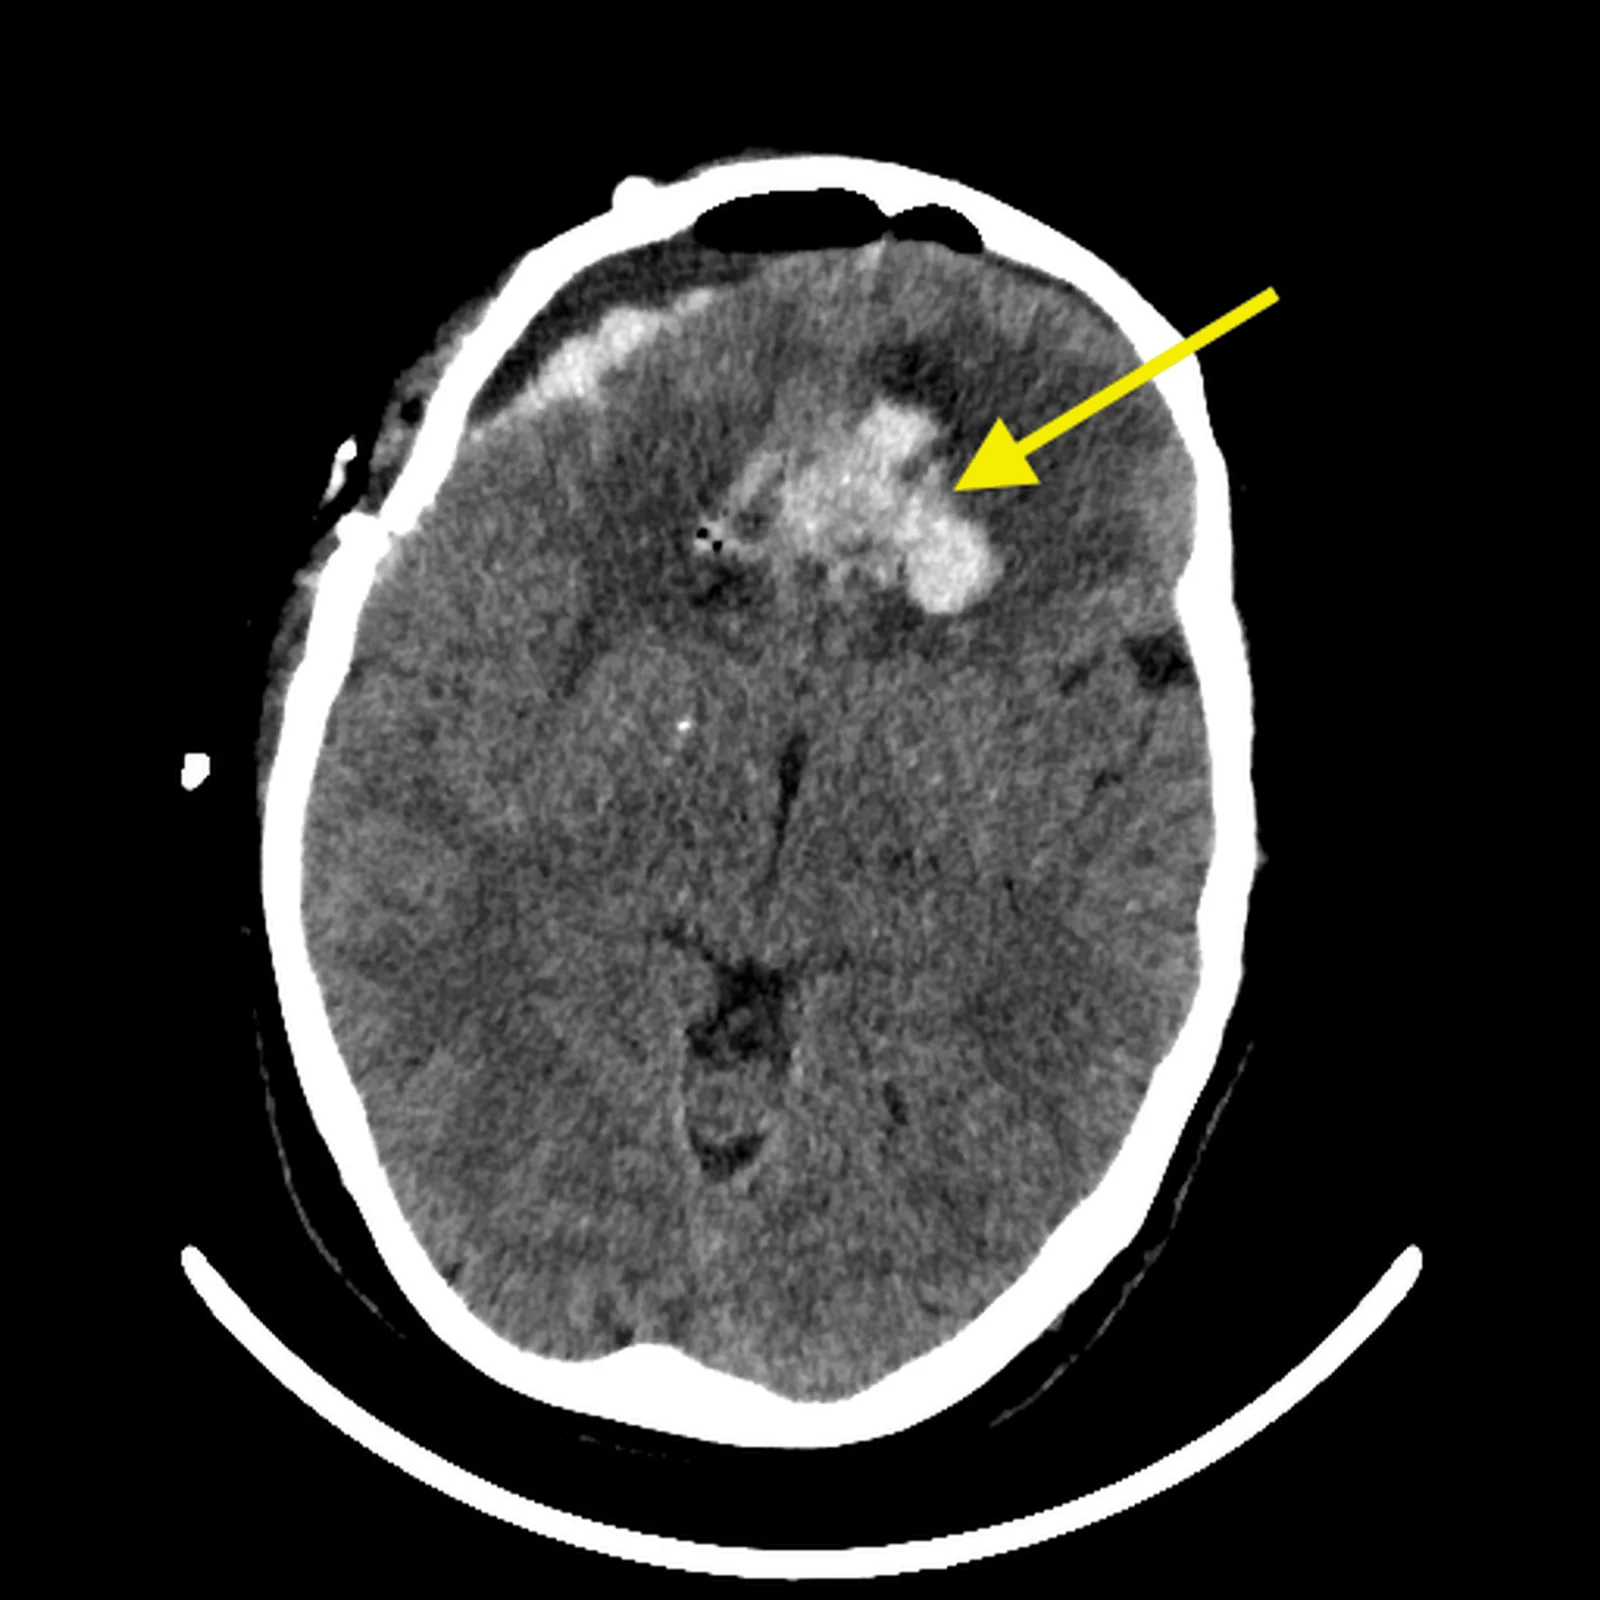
Postoperative non-contrast CT obtained on day 1 Axial CT image demonstrating a hematoma within the surgical cavity following right fronto-orbital craniotomy, without significant mass effect or relevant midline shift. The solid arrow indicates the postoperative hematoma CT: computed tomography
